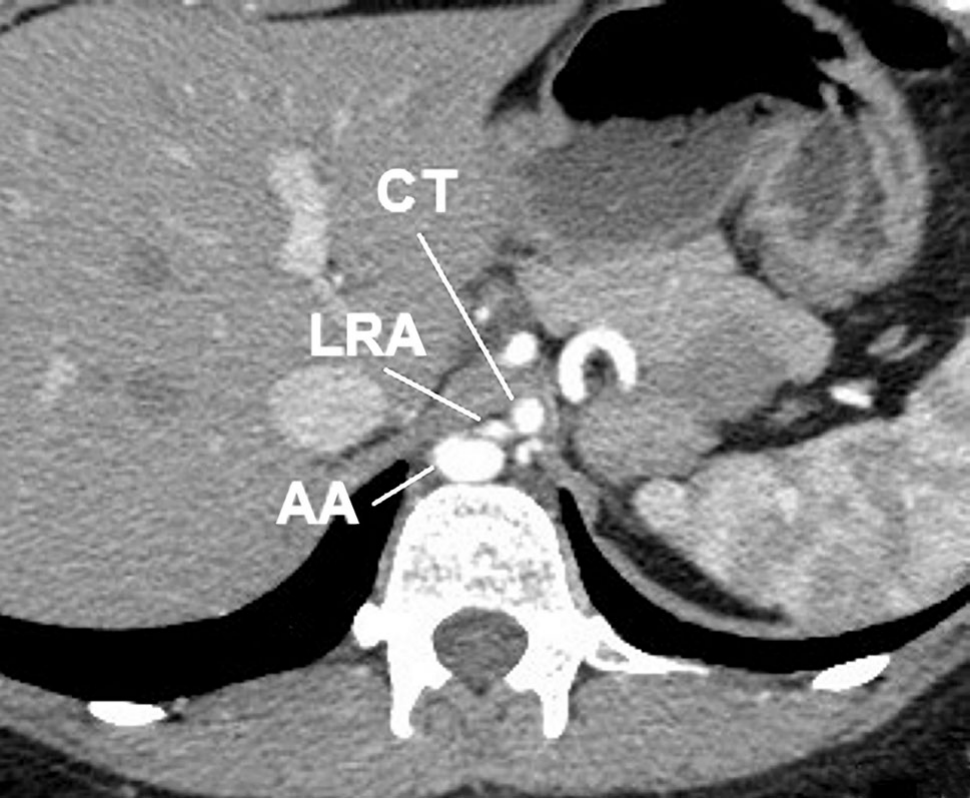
Abdominal computed tomography angiography, transverse scan. *AA* abdominal aorta, *CT* celiac trunk, *LRA* left renal artery
